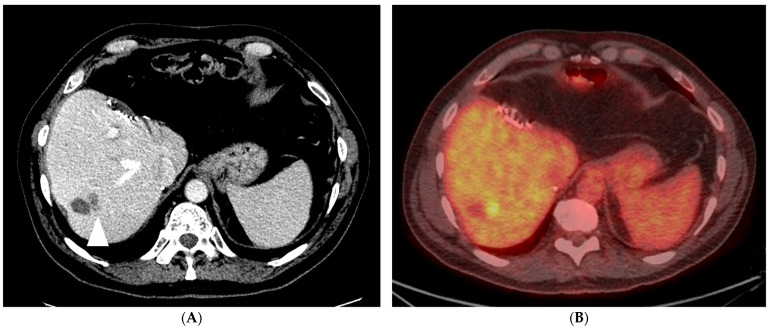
A 67-year-old man with metastatic rectosigmoid cancer, with imaging consistent with local tumor progression, one year after microwave ablation of segment 7 metastasis. Portal venous phase CT shows 1.3 cm hypoattenuating (relative to liver parenchyma) nodule along the medial aspect of the ablation zone ((A); arrowhead), with corresponding increased focal FDG-uptake on PET/CT (B). The recurrence was treated with repeat microwave ablation.
